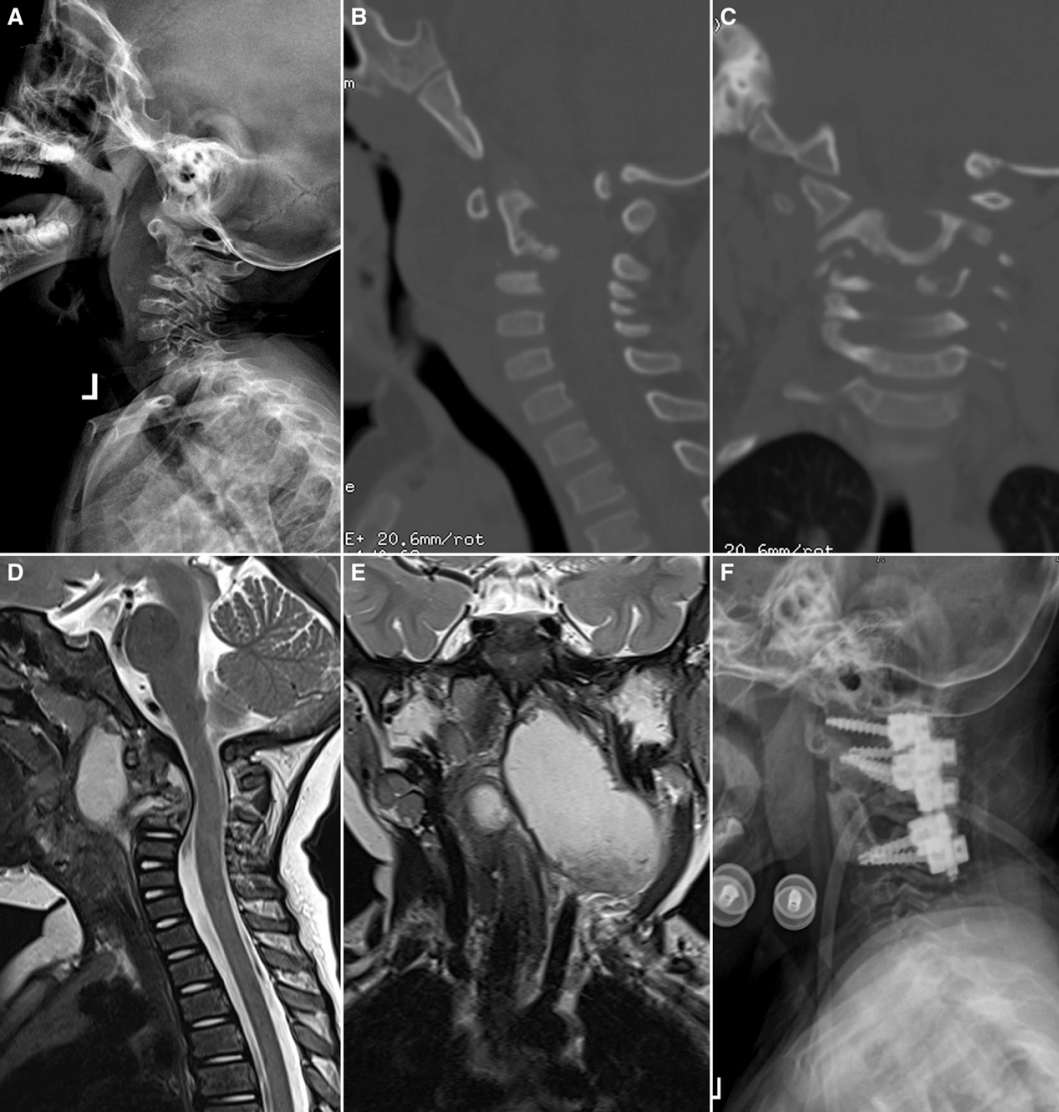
(A) Preoperative cervical plain radiograph. (B and C) Computed tomography scans indicated bone destruction of C2 and C3 vertebrae. (D and E) Magnetic resonance imaging showed a massive paraspinal abscess. (F) Postoperative cervical plain radiograph.
